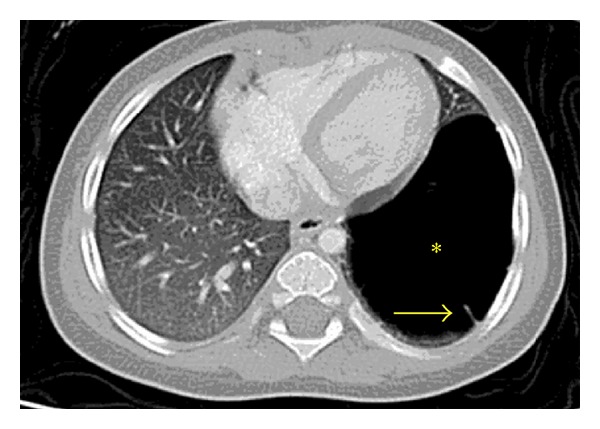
Axial lung window CT image shows a large thin walled cyst (asterisk) with a single septation (arrow) in the left lower lobe that is proved to be a type I PPB.
